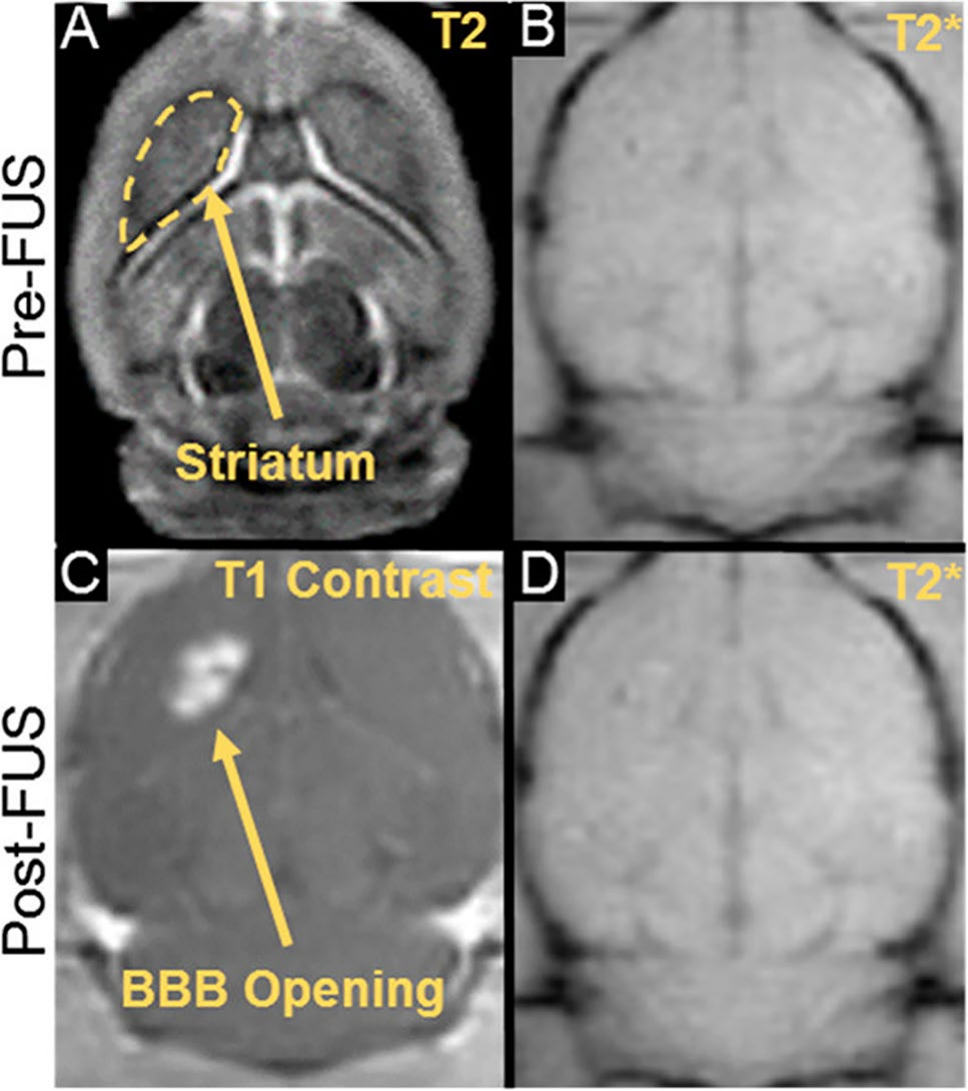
MR imaging for guidance, confirmation, and safety evaluation of FUS treatments. (A) Pre- treatment planning using T2 pre-FUS images. (C) BBB opening in the striatum as confirmed by post-FUS contrast-enhanced T1 imaging. (B, D) Treatment safety may be assessed by comparing pre- and post-FUS T2* images. Adapted from Mead et al. (2017). Reproduced with permission.
